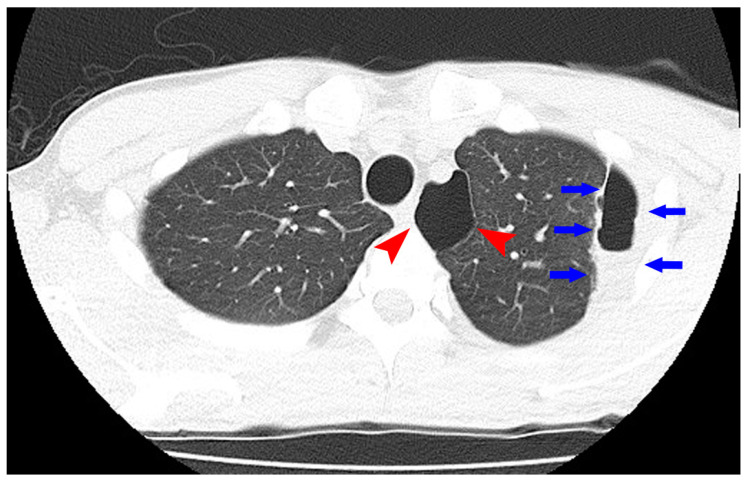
Computed tomography of the chest showed an emphysematous bulla (Arrowhead) in the upper left lobe of the lung and fluid formation inside the bulla (Arrow).
